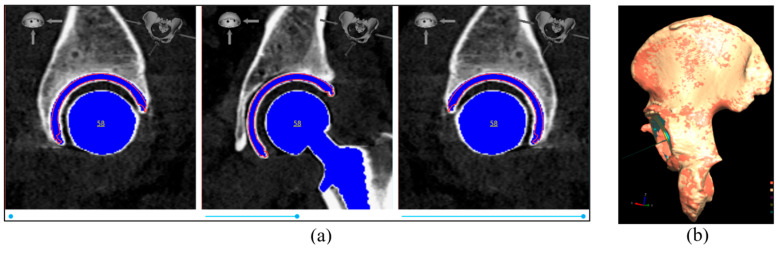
(a) Example of cup placement on the post-op CT image; (b) example of pelvis bone alignment from post-op scan (orange color) to pre-op scan (red color). In this example, the alignment accuracy (average surface distance between the two pelvis bones) is 0.162 mm.
